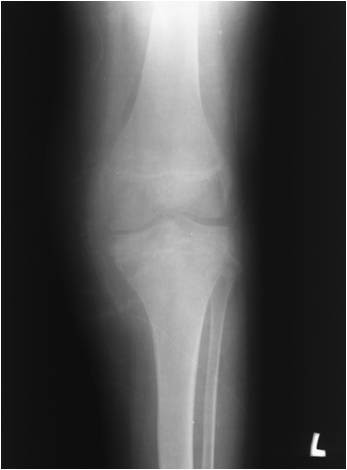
Radiograph of the affected knee with an osteolytic lesion in the proximal tibia.
